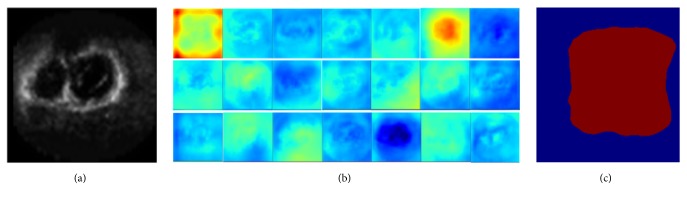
The feature maps of the last layer of FCN model with pretrained weights on VOC datasets, adopting the 2D echocardiography as input. (a) The original input 2D slice. (b) 21 feature mappings on the last layer. (c) The final output result of FCN.
